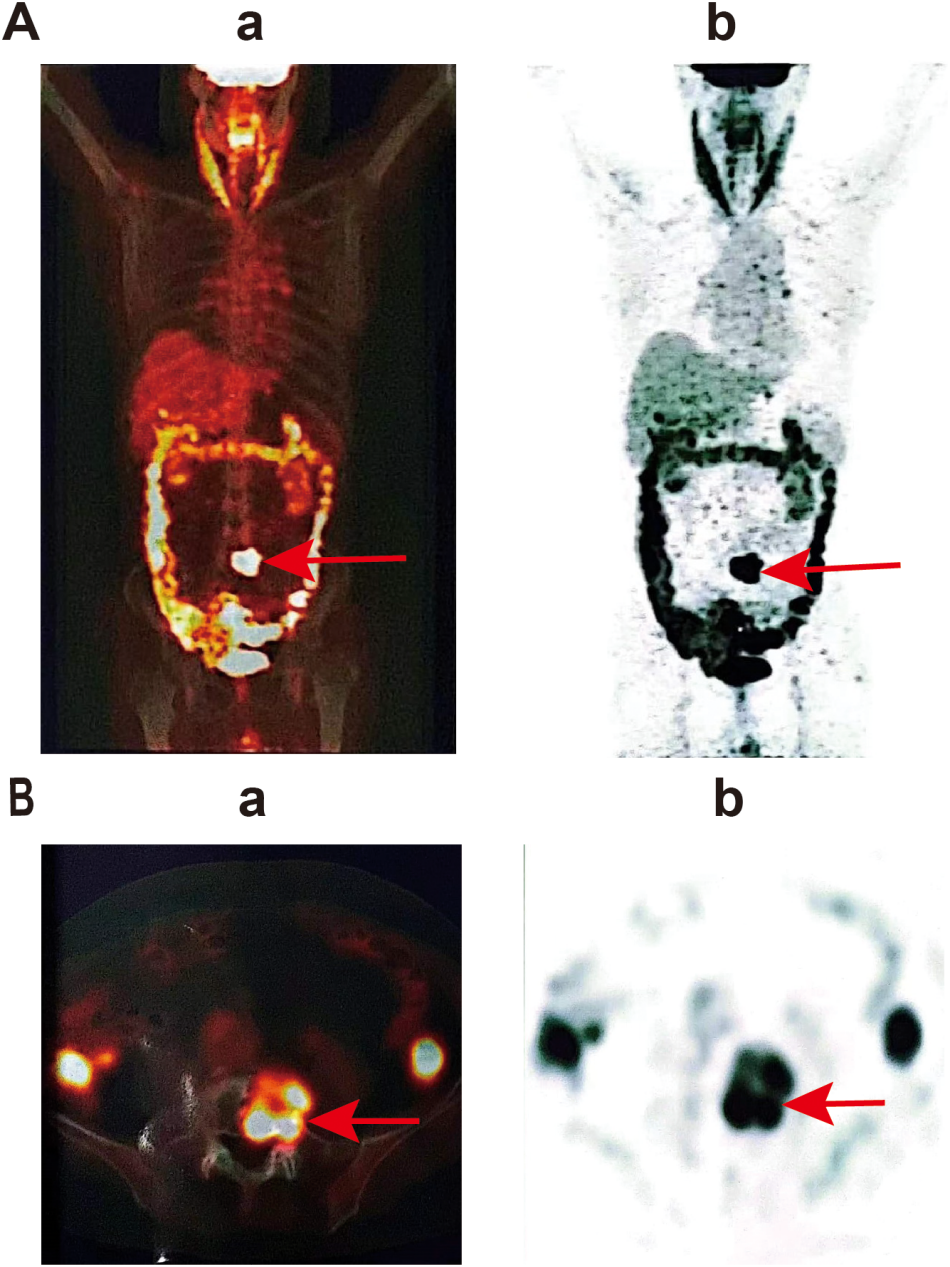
PET-CT examination showed the lesion in L5. (a) Positron emission tomography image, (b) computed tomography image. (A) represents a whole-body scan image, showing the overall metabolic activity of the patient; (B) is a local scan image, which is a transverse view, and the area pointed to by the red arrow shows the high metabolic activity in the local region.
